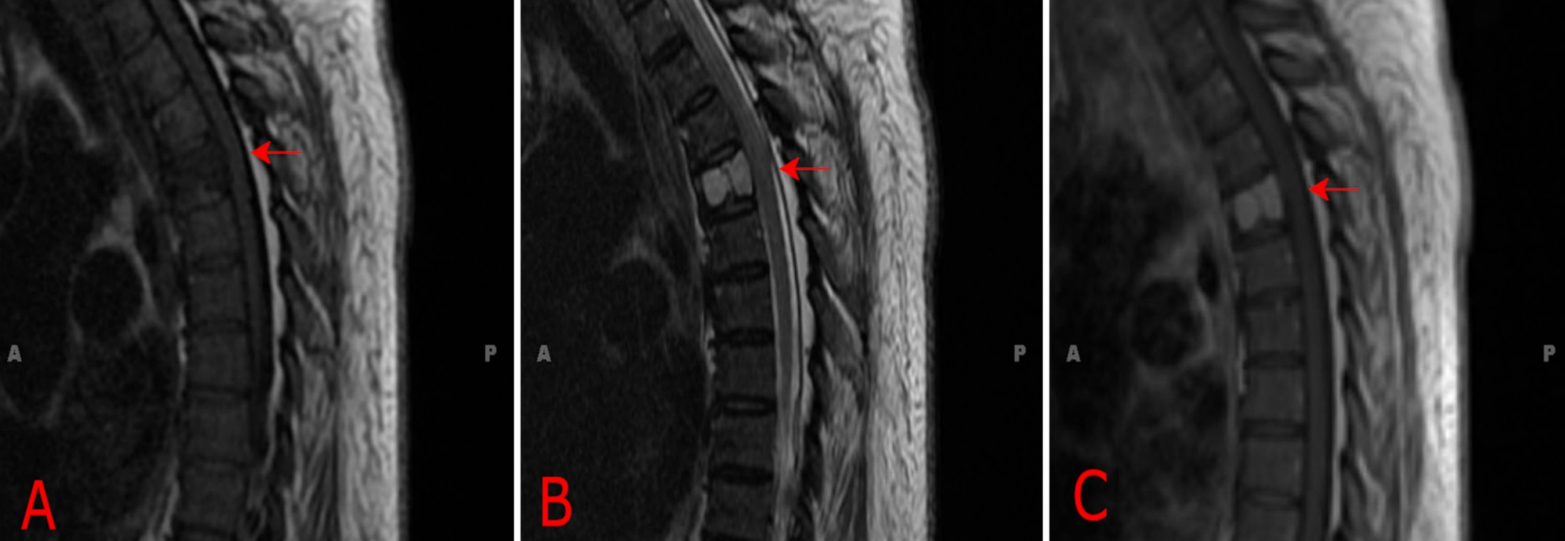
T1-weighted MRI displaying hypointense lesion (A), T2-weighted MRI displaying hyperintense T4 lesion (B), and T1-weighted MRI with contrast displaying enhancement (C) MRI (Magnetic Resonance Imaging)
